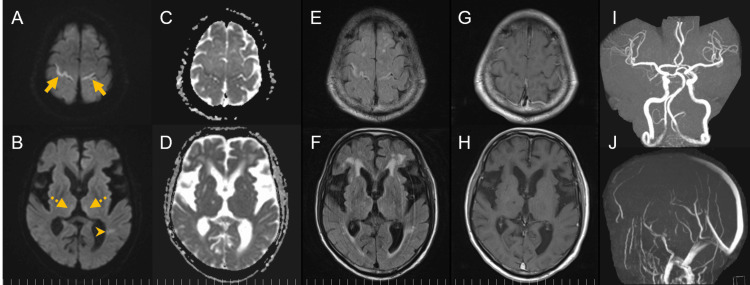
Brain MRI during altered mental status (A, C, E, G) Prominent cortical ribboning lesions (arrows) are observed in both frontal cortices, showing high signal intensity on DWI, reduced ADC, and high signal on FLAIR, with no gadolinium enhancement. (B, D, F, H) Less prominent lesions (dashed arrows) are observed in the medial thalami bilaterally, showing a similar pattern. The previously noted left parietal subcortical lesion (arrowhead), which had been identified a few days earlier, shows an unchanged high signal on DWI and FLAIR, but the reduction in ADC has resolved, suggesting that it represents a time-dependent change in cerebral ischemia. (I, J) Magnetic resonance angiography/venography showed no arterial or venous occlusions. ADC, apparent diffusion coefficient; DWI, diffusion-weighted imaging; FLAIR, fluid-attenuated inversion recovery
